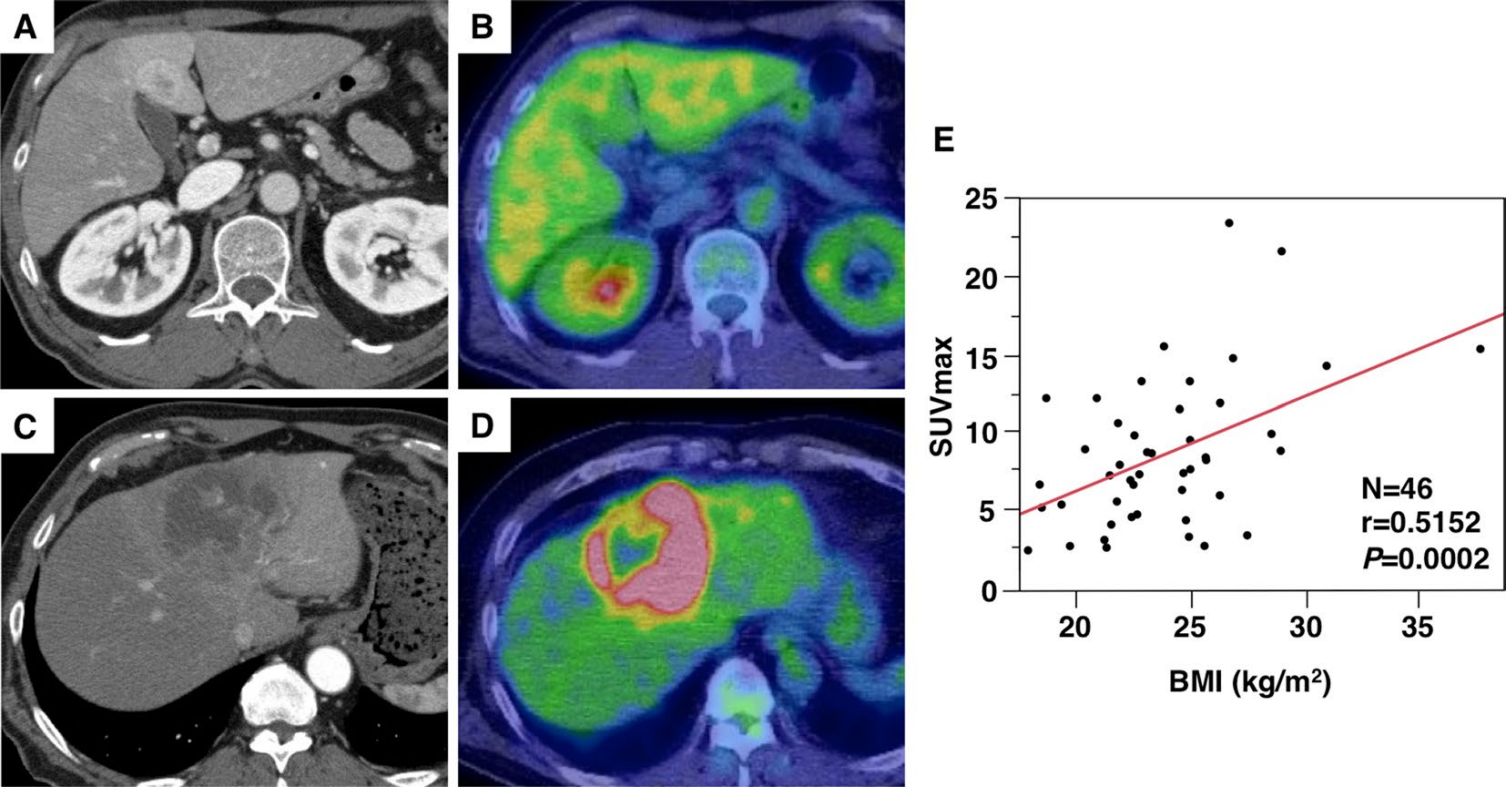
Representative positron emission tomography/computed tomography (PET/CT) images and the relationship between body mass index (BMI) and the maximum standardized uptake value (SUVmax) (n = 46). A patient with low SUVmax (< 6.79) on preoperative enhanced CT (A) and fluorine-18 fluorodeoxyglucose (^18^F-FDG) PET/CT (B). A patient with high SUVmax (≥ 6.79) on enhanced CT (C) and ^18^F-FDG PET/CT (D). The relationship between BMI and SUVmax (E).
